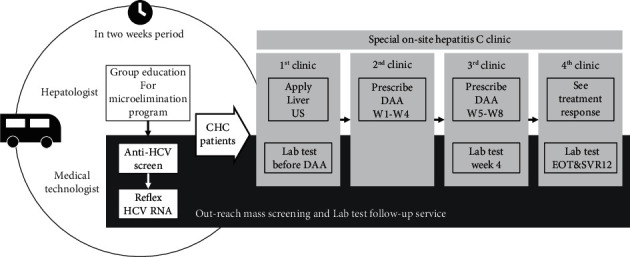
The on-site integrated screening and microelimination program in a detection center. Abbreviations: HCV: hepatitis C virus; CHC: chronic hepatitis C; US: ultrasound; Lab: laboratory; DAA: direct antiviral agent; EOT: end of treatment; SVR: sustained viral response.
